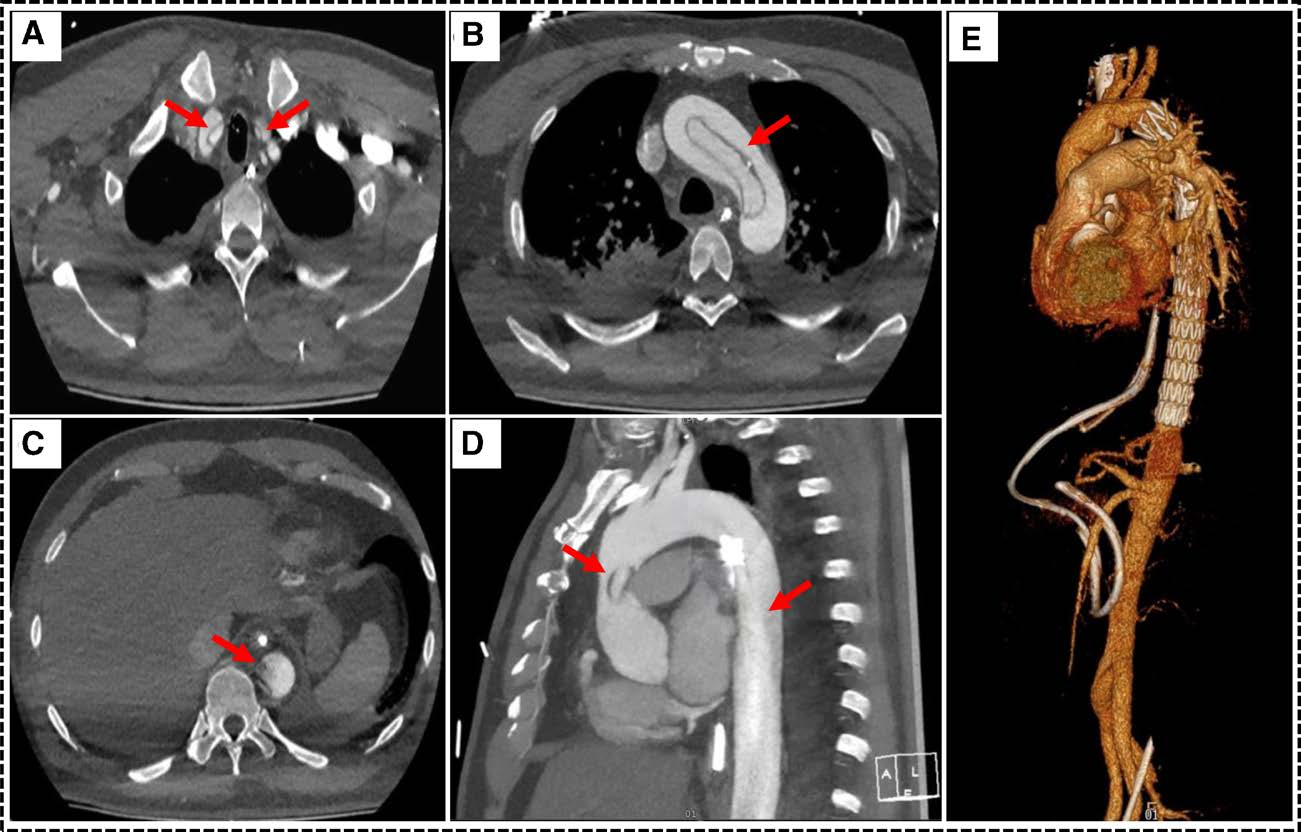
Preoperative vascular CTA. (A) Involvement into the innominate artery and the left common carotid artery (red arrows); (B) Involvement into the aortic arch (red arrows); (C) Involvement into the abdominal aorta, and the small-sized true-lumen (red arrows); (D) Involvement into the entire aorta (red arrows); (E) Three-dimensional reconstruction after type A aortic dissection surgery. CTA = computed tomography angiography.
